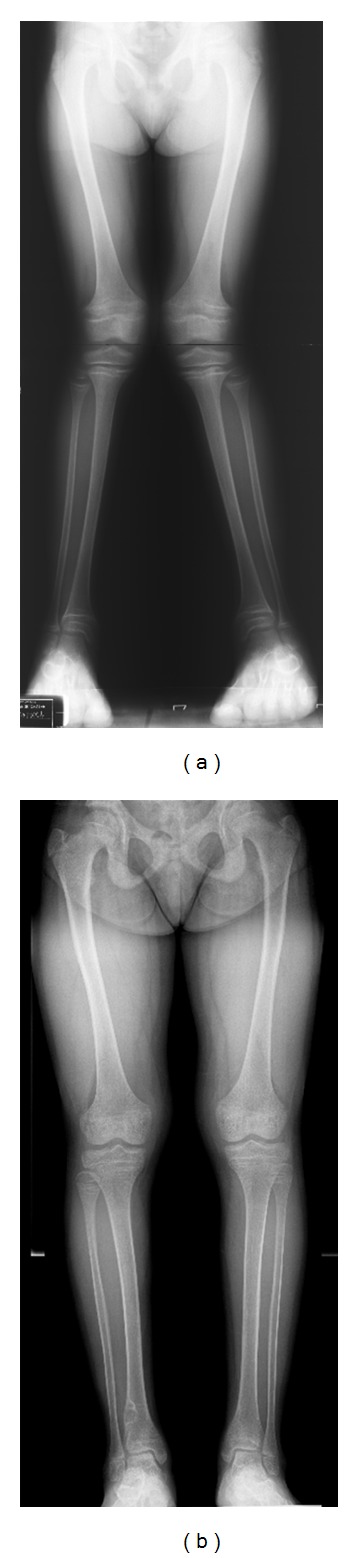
Radiograph of both lower extremities in a standing view. (a) Marked genu valgum deformity (24-degree angulation on the left and 20 degrees on the right) was noted at 5 years of age before phosphate and calcitriol therapy was commenced. (b) Marked improvement in genu valgum and healing of rickets at 10 years of age (4-degree angulation on the left and 5 degrees on the right).
